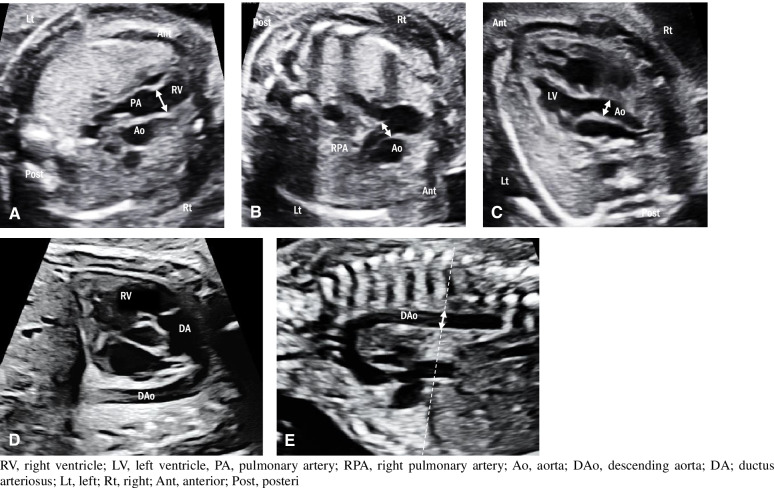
Measurements of the fetal cardiac parameters. In the right ventricular outflow tract view, pulmonary valve annulus is measured from hinge point to hinge point at the location of maximal expansion during peak systole (A, arrow). In the three-vessel view, the right pulmonary artery is measured at the proximal part of its bifurcation (B, arrow). In the left ventricular outflow tract view, the aortic valve annulus is measured from hinge point to hinge point at the location of maximal expansion during peak systole (C, arrow). In the ductal arch view, pulmonary valve peak systolic velocity is measured at the level of the pulmonary valve using pulsed Doppler (D). In the aortic arch view, the descending aorta is measured at the level of the diaphragm (E, arrow)
